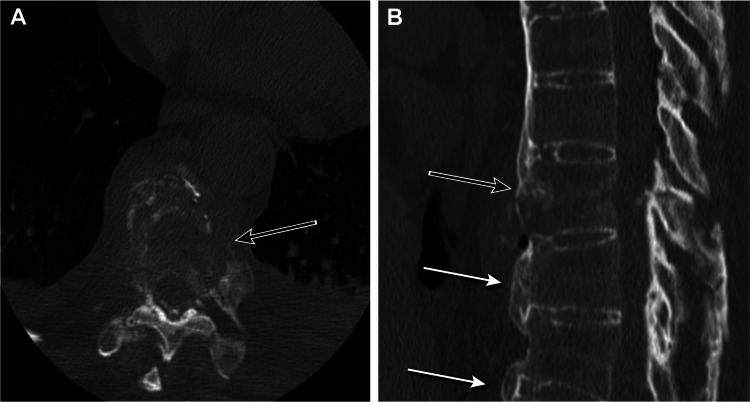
Preoperative computed tomography of the lumbar spine in case 2. Axial (A) and sagittal (B) preoperative imaging showing typical signs of ankylosing spondylitis (white arrows) as well as a T9 burst fracture (black arrows). Used with permission from Barrow Neurological Institute, Phoenix, Arizona.
